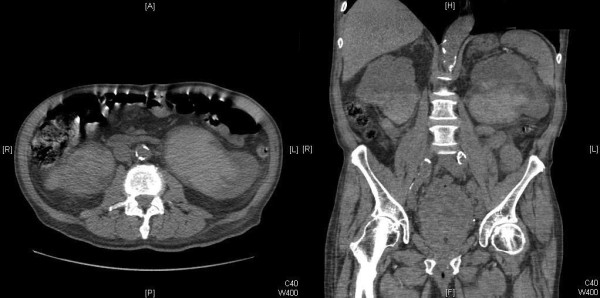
Computed tomography scan confirming ultrasound findings.
